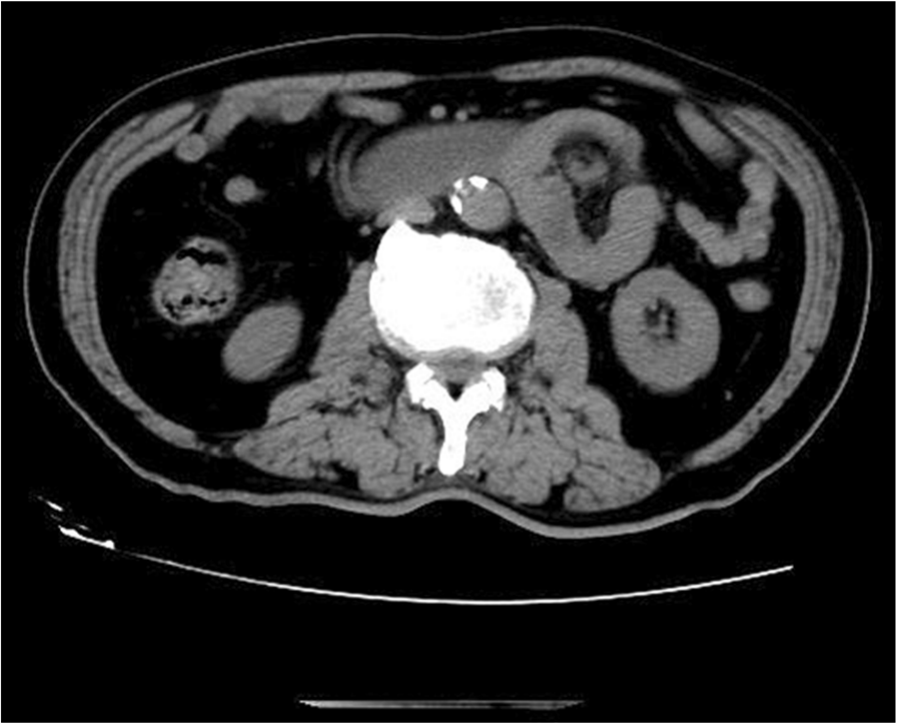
Transverse section of CT scan image showing the cluster of jejunum
